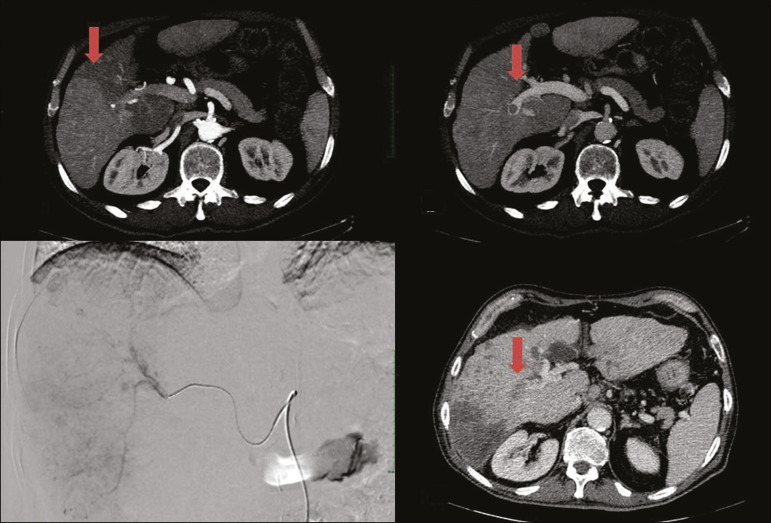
DSM-TACE of multi-nodular HCC. Contrast-enhanced computed tomography scan of a 75-year-old patient with liver cirrhosis and a multi-nodular HCC involving the right liver lobe, showing enhancement in the arterial phase (arrow). Note the right portal vein thrombosis (arrow). Right hepatic artery digital subtraction angiography showing the multi-nodular hypervascular lesion. Follow-up contrast-enhanced computed tomography scan, acquired one month later, demonstrating a partial response, with tumour necrosis and a thrombus remaining in the right branch of the portal vein (arrow).
